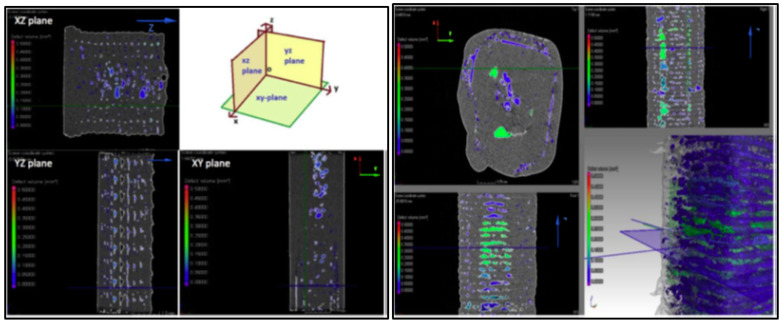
Computed Tomography scans of gauge length of PEEK-XY-100 (left) and PEEK-Z-100 (right) samples before tensile test [85].
